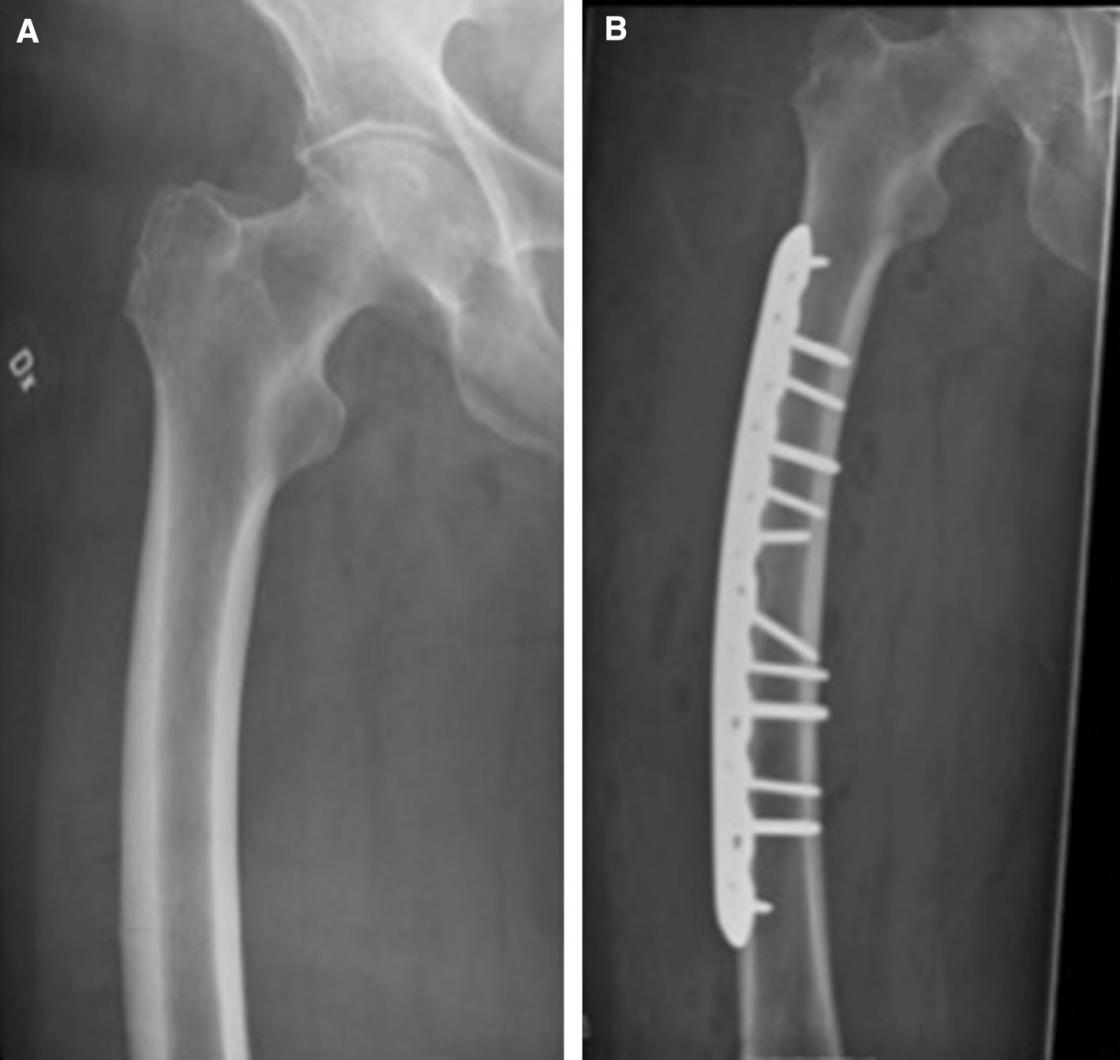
a An 80-year-old female sustained an incomplete fracture of her right femur. Before the fracture, she had received 5 years of treatment with alendronic acid because of a high dose of corticosteroids for rheumatic disease. She recalled enduring 6 months of increasing pain from her right thigh before seeking medical advice. b Surgery was selected as the preventive treatment of choice. Bisphosphonate treatment was discontinued before surgery. Lateral fixation was performed because of the curvature of the femur (femoral angle approximately 10°). Full weight bearing was allowed postoperatively. Radiographic examination after surgery revealed no further propagation of the fracture
